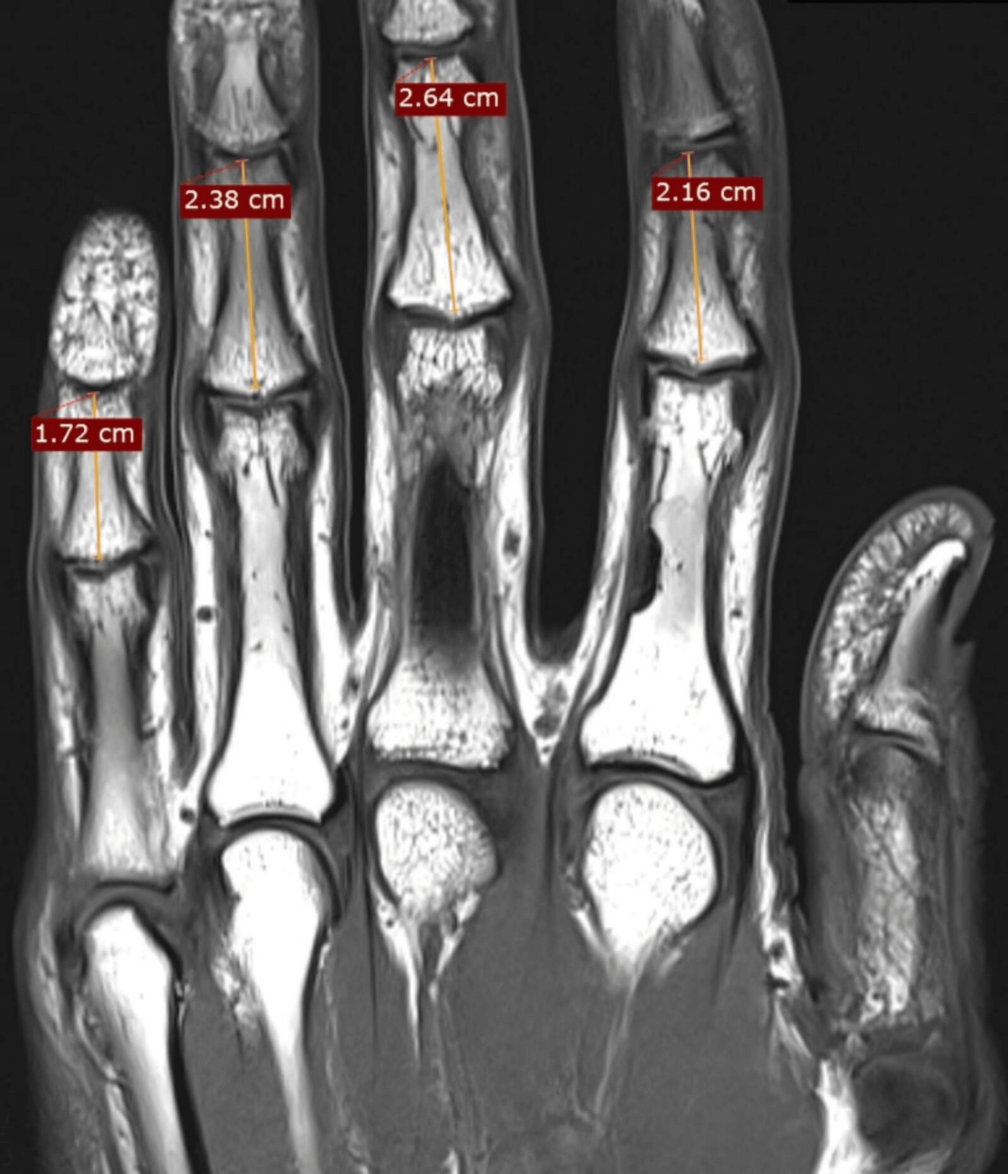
Technique showing the measurement of the length of the middle phalanx on coronal T1-weighted MRI sequences.
